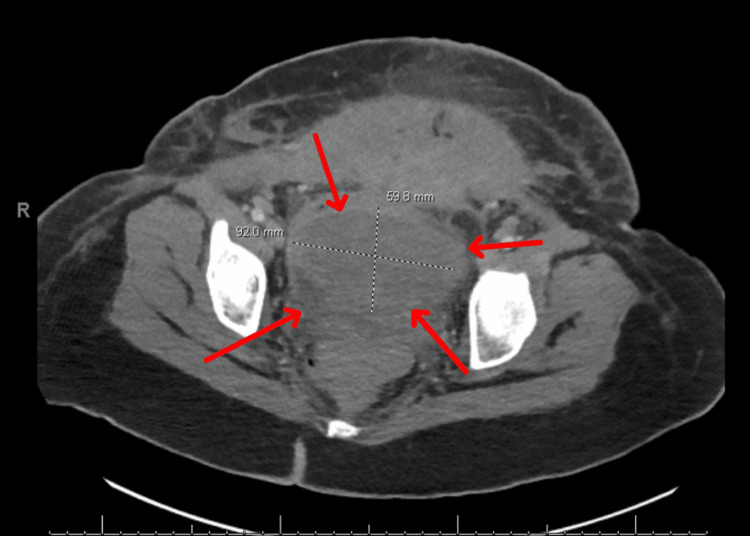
CT abdomen and pelvis. There is a 9 x 6 cm hematoma (red arrows) superior to the bladder. CT: computed tomography
